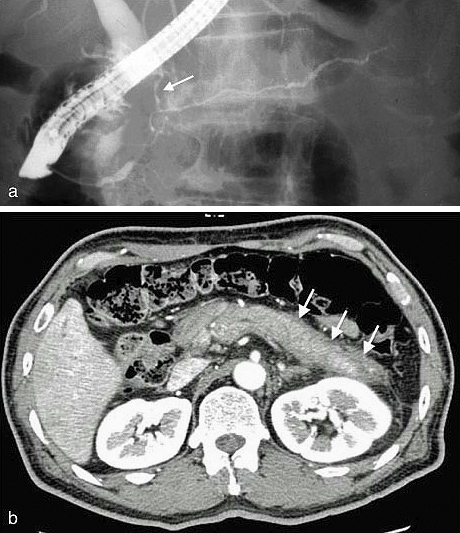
Image findings of AIP. (a) Endoscopic retrograde cholangiopancreatography (ERCP) typically shows characteristic irregular narrowing of the main pancreatic duct and narrowing of the lower bile duct (arrow). (b) Contrast-enhanced CT reveals a swollen pancreas with a straight margin and capsule-like, low-density rim (arrows). (From Shindan to Chiryo 104;453–458:2016 in a Japanese publication. Reprinted with permission from Igakutosho-shuppan, Ltd.)
